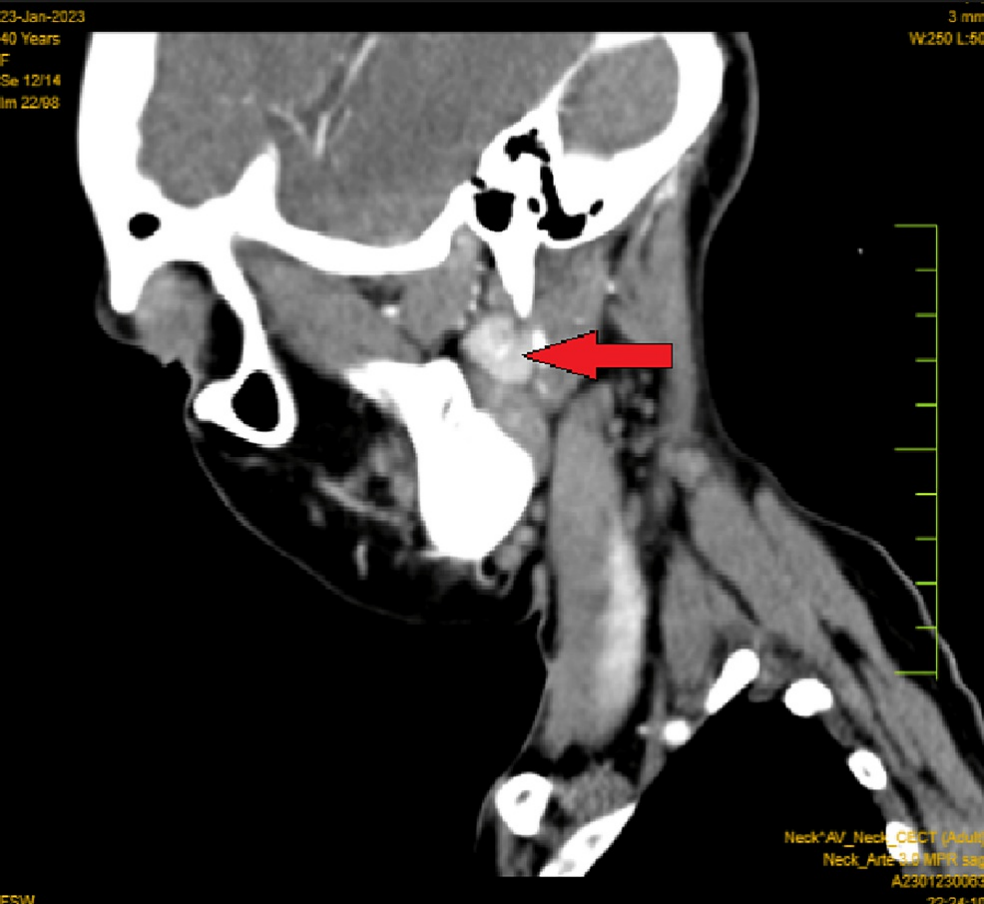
CT Head and Neck (Sagittal section)
